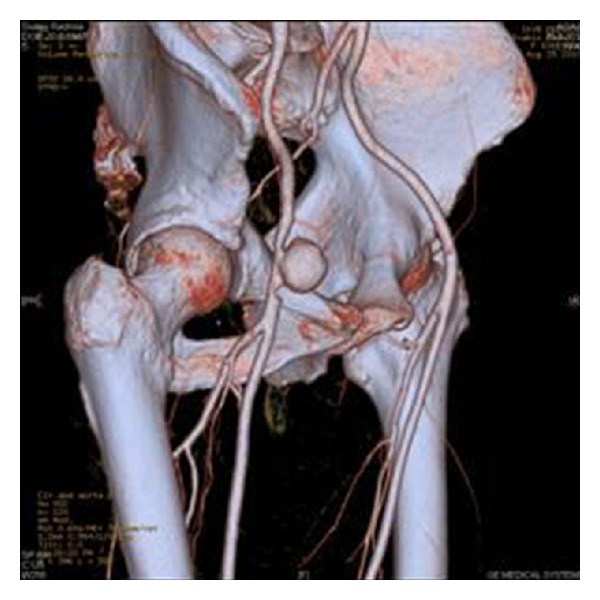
Pseudoaneurysm of right common femoral artery on CT angiography.
